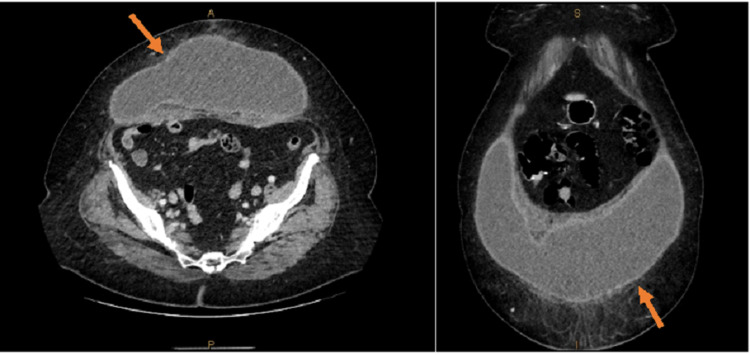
CT abdomen (axial slice on left, sagittal slice on right) taken a year after hernia repair with chronic seroma (orange arrows).
